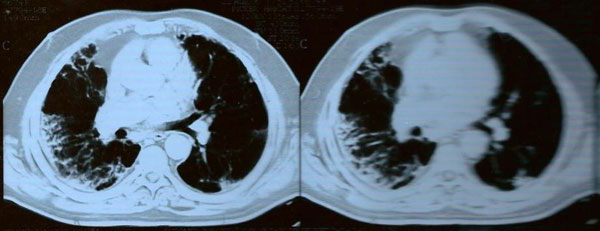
High-resolution computed tomography of the chest (HRCT) demonstrates bilateral interstitial infiltrates, predominantly in the lung bases and periphery with subpleural parenchymal bands and ground-glass opacities that were greater on the right than on the left consistent with interstitial pneumonitis.
